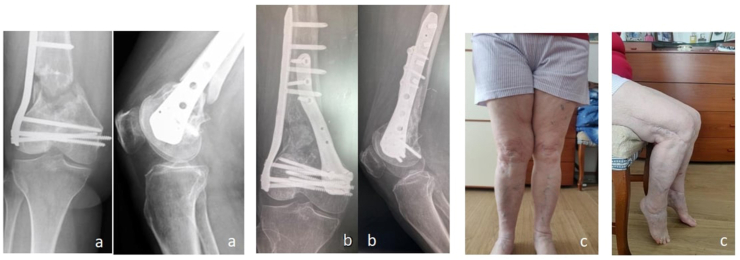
Clinical case n°2. Pre-op X-rays (a), Post-op x-rays (b) and clinical (c) results at 15 months.
